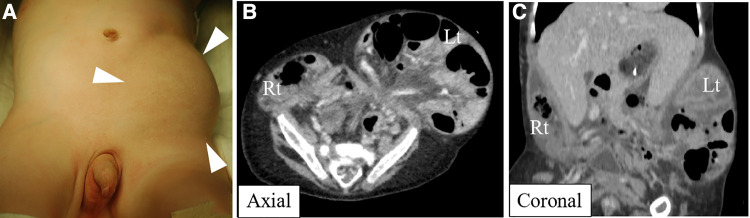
Physical examination revealed left flank swelling (A), and computed tomography revealed a bilateral inguinal hernia extending into both flanks between the abdominal wall and the skin (B,C).
